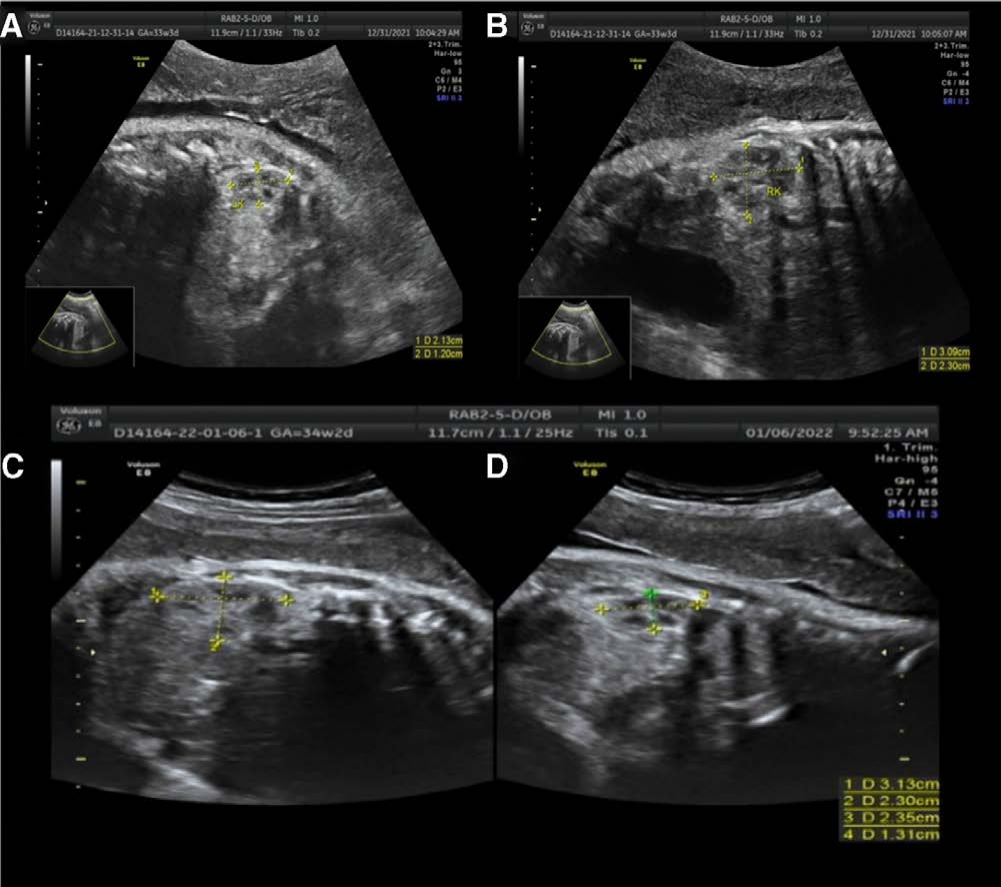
Ultrasound image of fetal kidney. A, B: Left and right kidney size at 33 weeks + 4 days; renal volume decreased and echo increased; C, D: 36 weeks + 3 days, the volume of both kidneys decreased and the echo increased.
